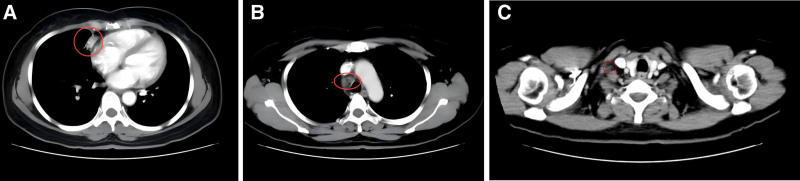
Chest CT follow-up on June 11, 2020. (A) Significant reduction in the right lung lesion; (B) further reduction in the size of the enlarged mediastinal lymph nodes; (C) further reduction in the size of the metastatic lymph node in the right supraclavicular region. CT = computed tomography.
